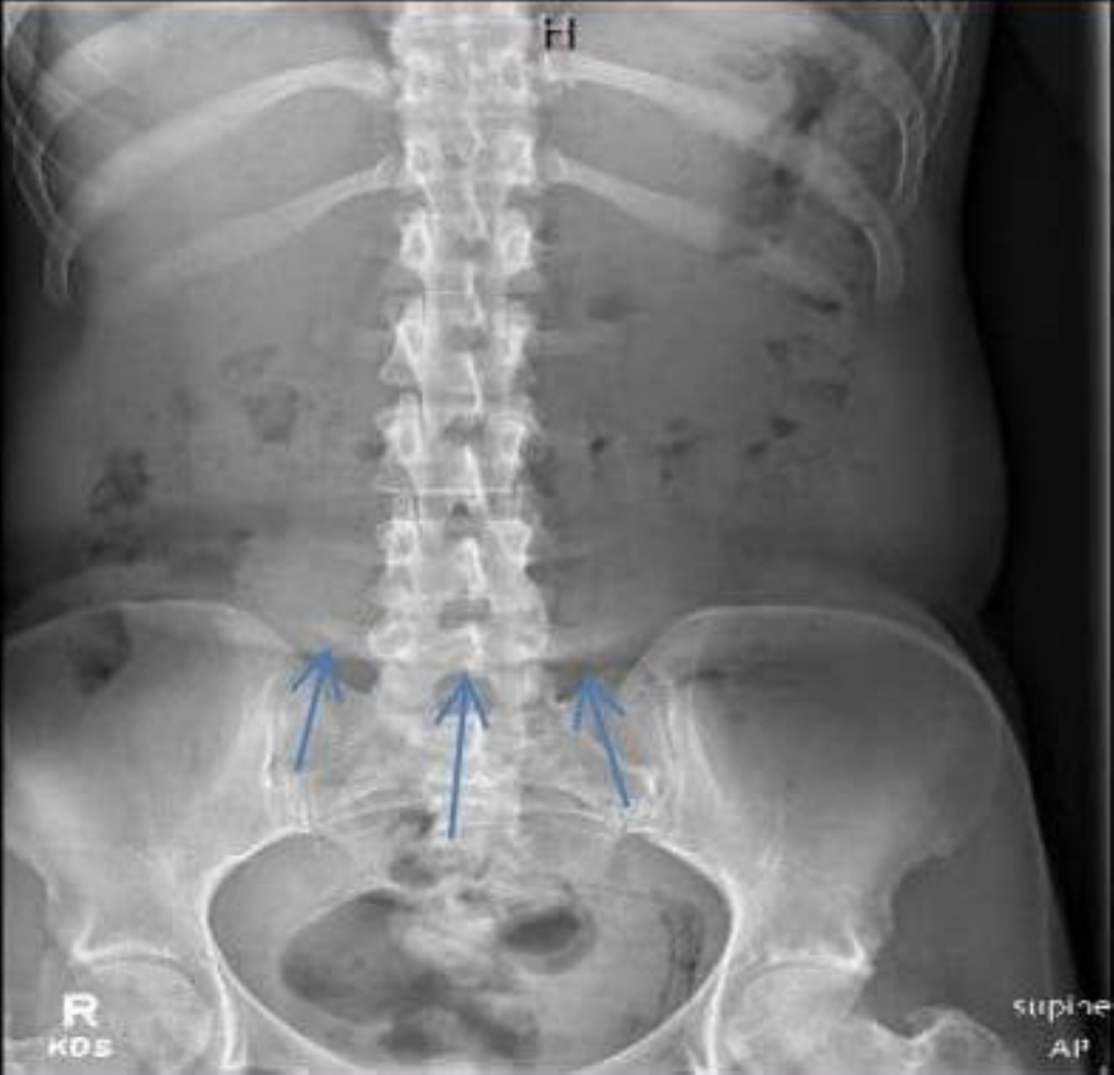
Abdominal X-ray demonstrating curvilinear lucencies in the pelvis
